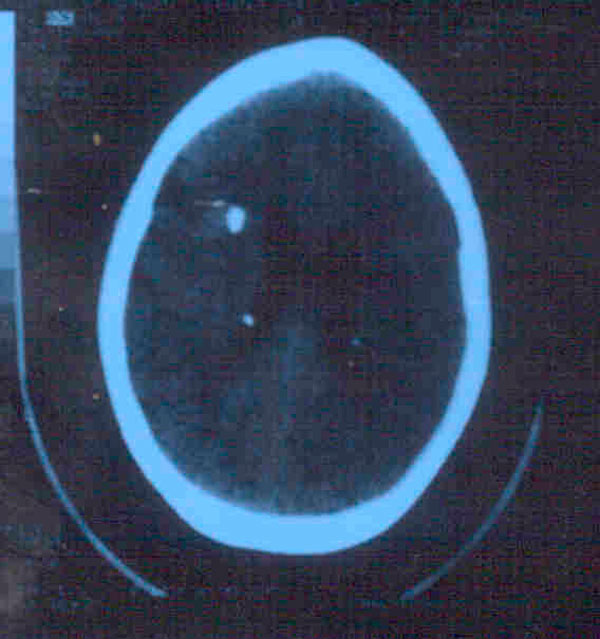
Computerized tomography (CT) of the brain showing bilateral subependymal periventricular tubers.
